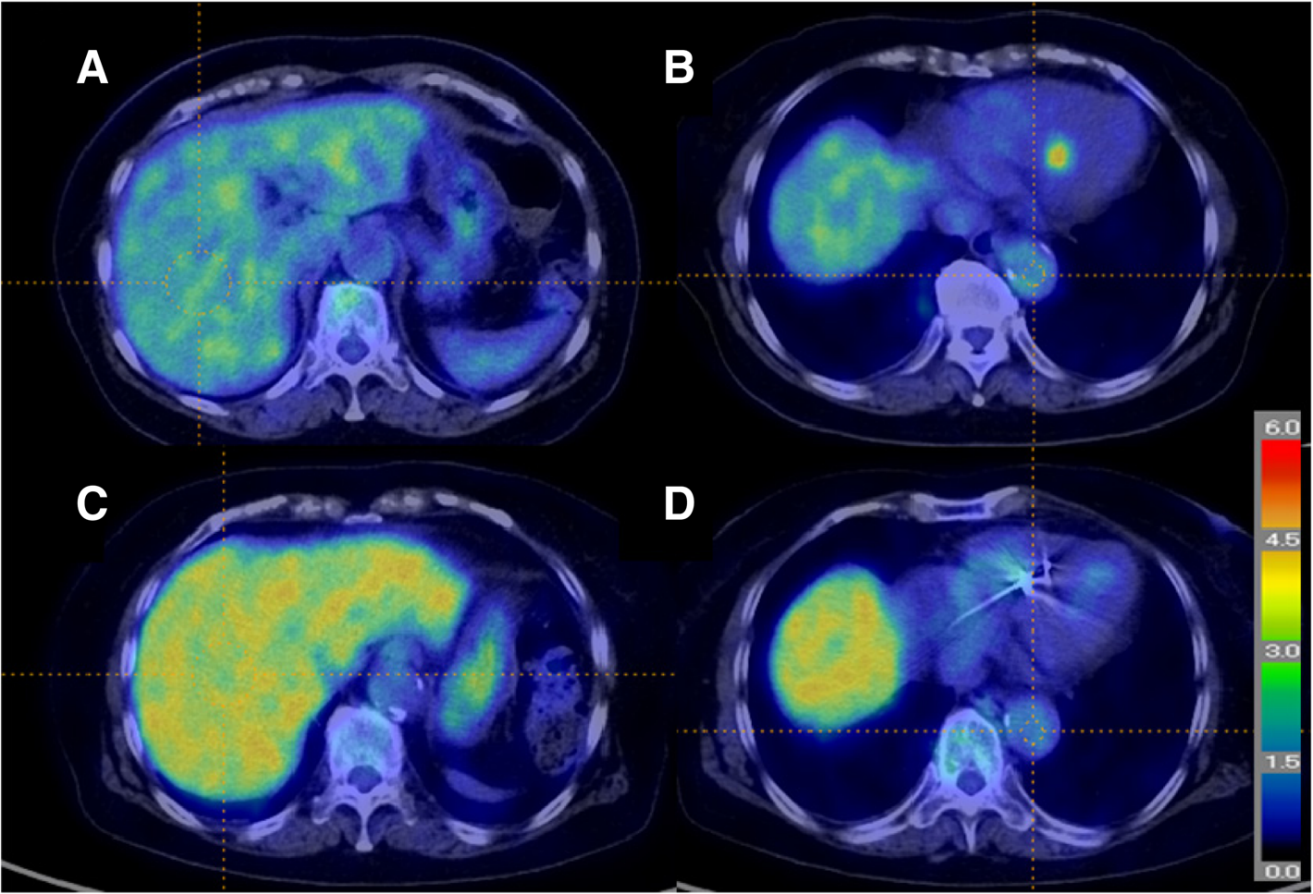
A representative case before and during the oral steroid therapy of CS. ^18^F-FDG PET/CT images obtained before (a, b) and during (c, d) treatment of CS. Note the profound decline in the myocardial uptake and increase in the liver uptake. The threshold increased from the time point before (a, 3.7) to that during steroid therapy (c, 4.5). The SUV of the DA showed little change from the time point before (b, SUVmax 2.9; SUVmean 2.6) to that during steroid therapy (d, SUVmax 2.8; SUVmean 2.5)
